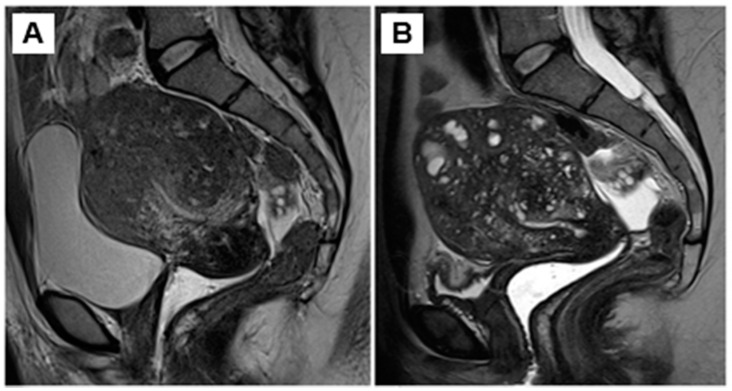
(A) MRI showing an enlarged uterus with diffuse and disseminated adenomyosis. (B) Worsening of MRI features after therapy with UPA. Numerous spots typical of adenomyosis are visible: asymmetric heterogeneous myometrium with multiple myometrial cysts, related to dilated islets of ectopic endometrium.
